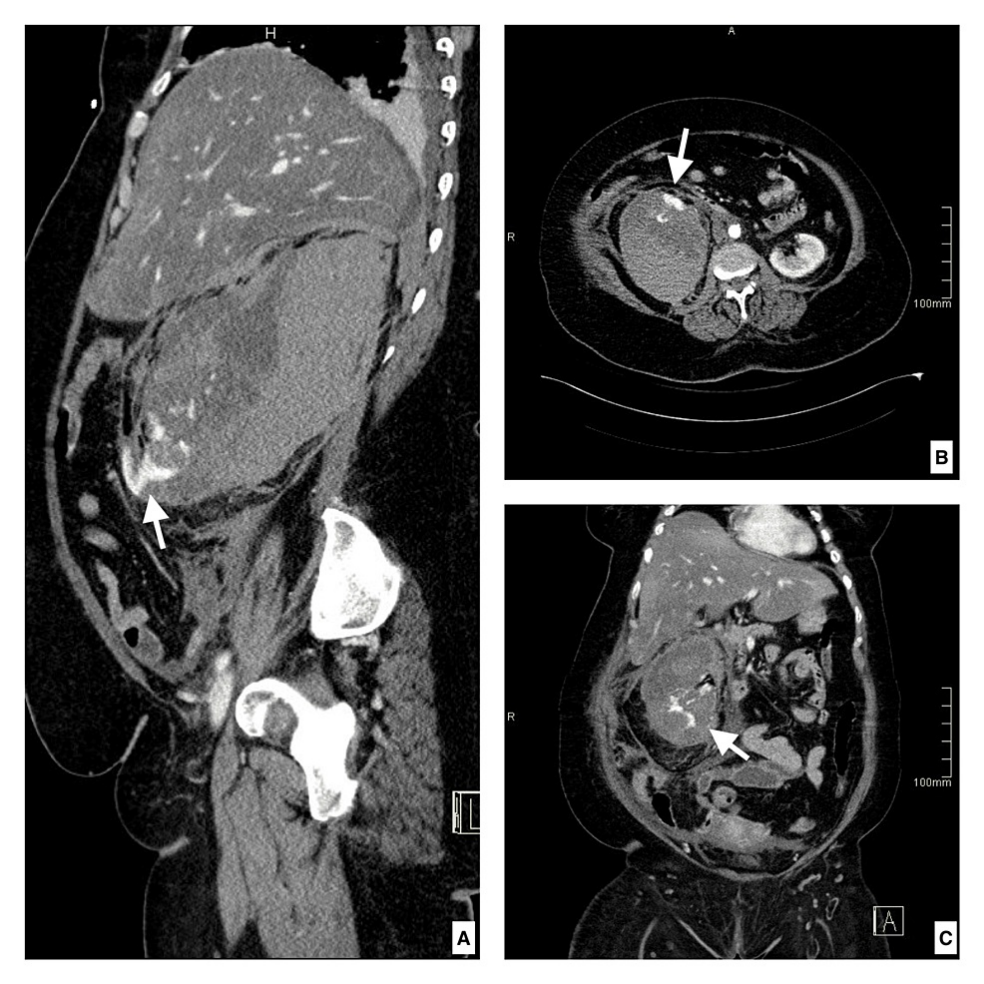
Enhanced sagittal, axial, and coronal CT scans acquired in the arterial phase Seen is a shattered kidney with cortical distortion and active contrast extravasation (white arrows in images A, B, and C).
